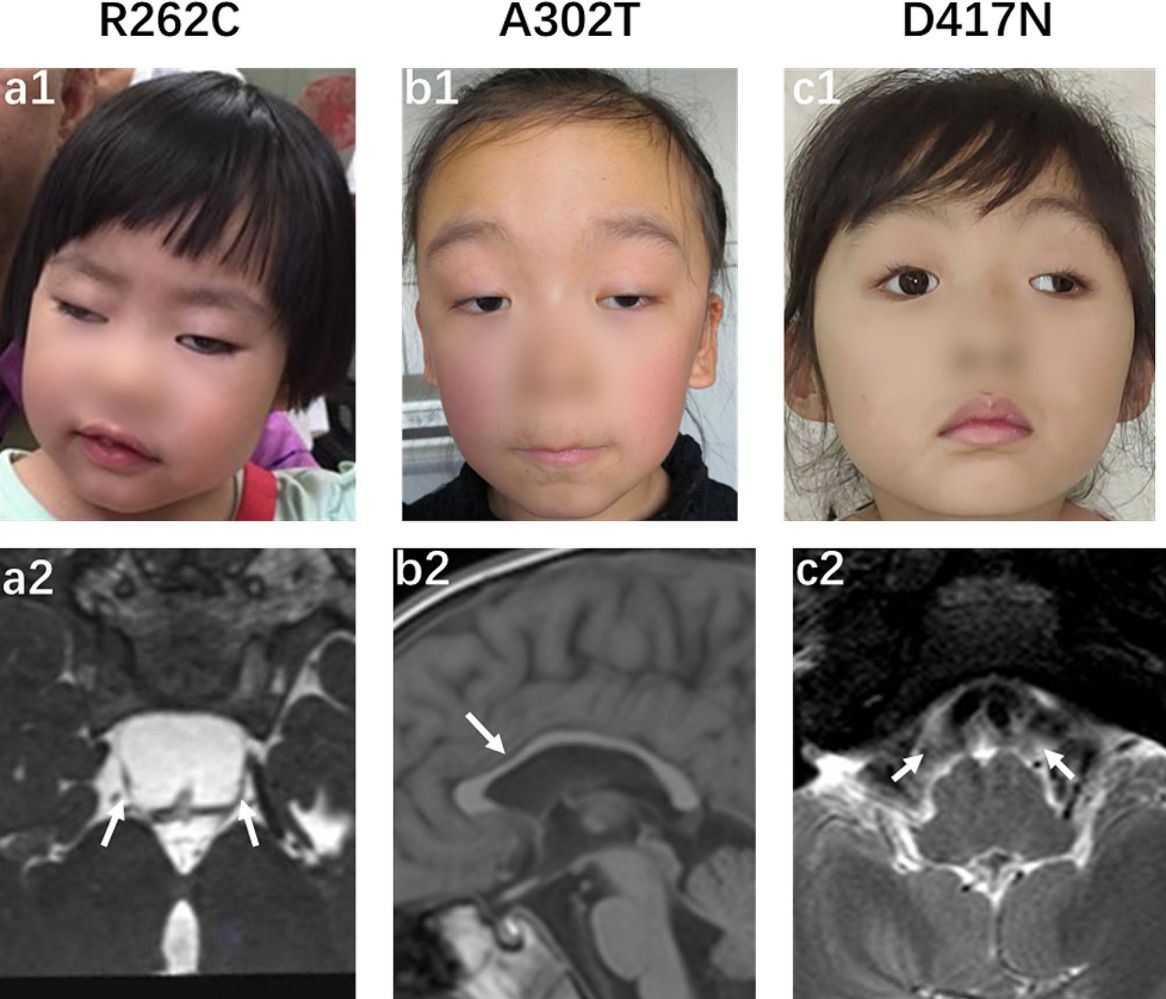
Ocular and MRI findings of patients with CFEOM3 carrying *TUBB3* variants. a1, a2 Patient P10-III1, harboring the Arg262Cys variant, displayed bilateral ptosis and hypoplasia of CN3 on MRI. b1, b2 Patient S21, harboring the Ala302Thr variant, showed bilateral ptosis and hypoplasia of the corpus callosum on MRI. c1, c2 Patient S24, harboring the Asp417Asn variant, exhibited left ptosis and absence of bilateral CN6 on MRI
